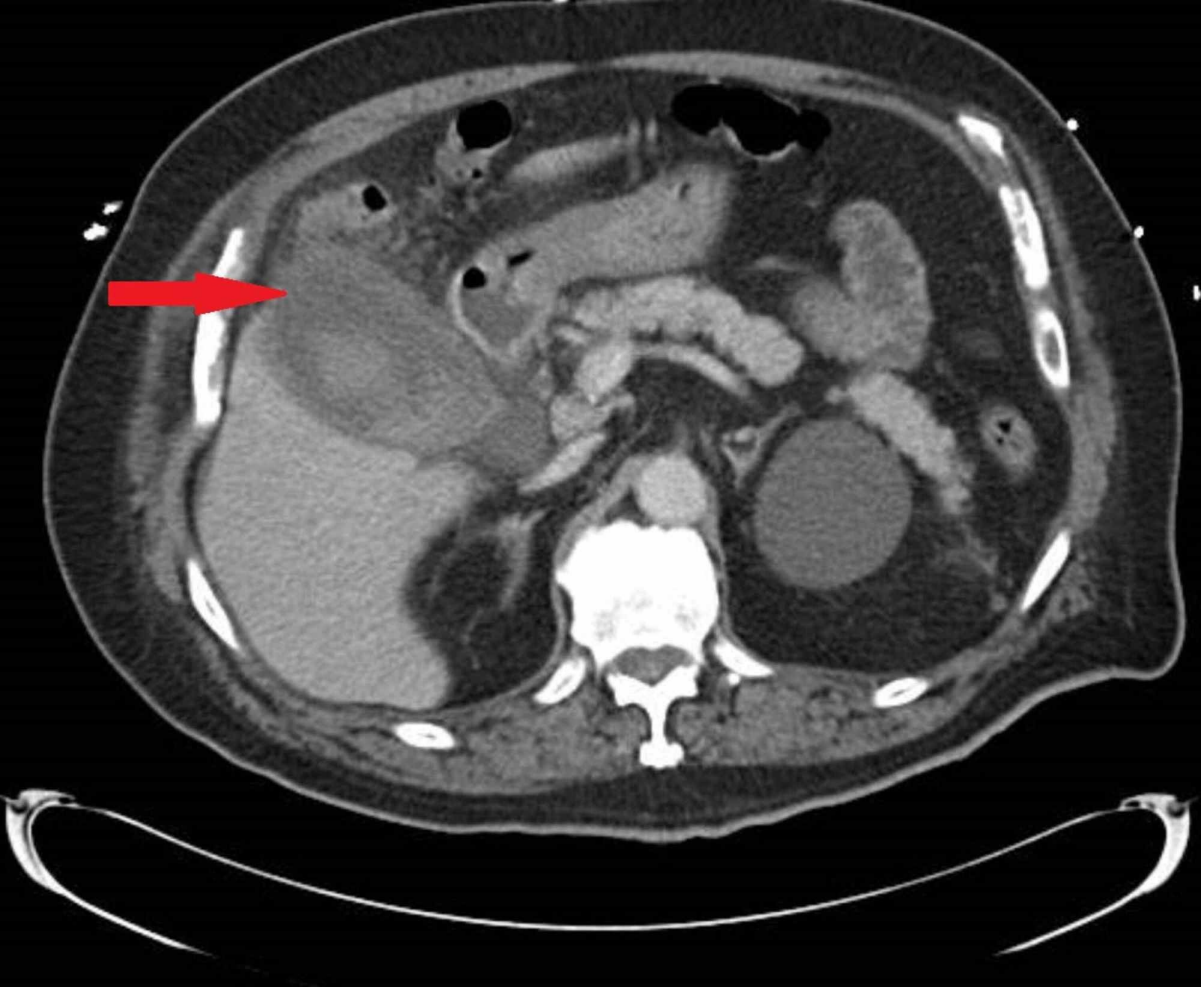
Computed tomography of the abdomen with contrast showing distended gallbladder with surrounding inflammatory changes
